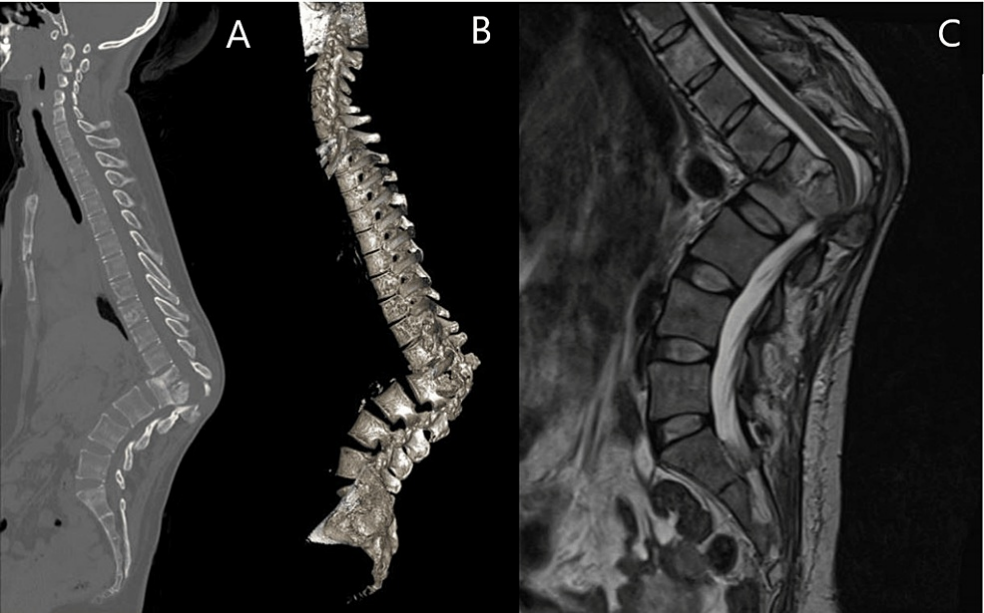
Preoperative CT (A) and 3D CT reconstruction (B) showing T12–L2 vertebrae with acute-angle kyphosis, and T2-weighted MRI (C) showing spondylodiscitis of the T12–L2 vertebrae with paraspinal collection.
